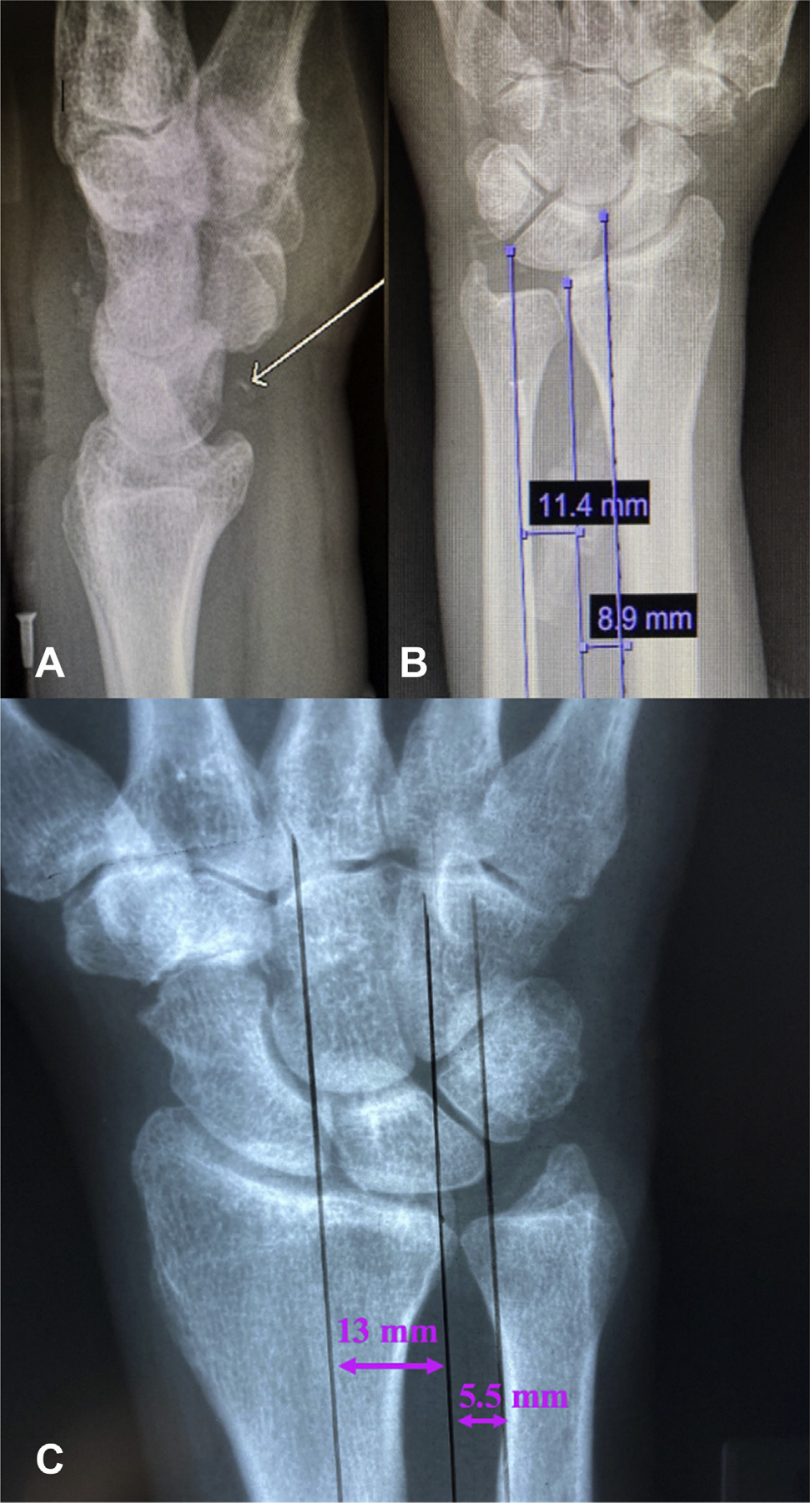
Plain radiographs of the injured and uninjured wrist. A Lateral view of the left wrist. Volar fleck is identified by the white arrow. B PA view of the left wrist. Lunate uncovering 56%. C PA view of the contralateral wrist. Lunate uncovering 30%.
